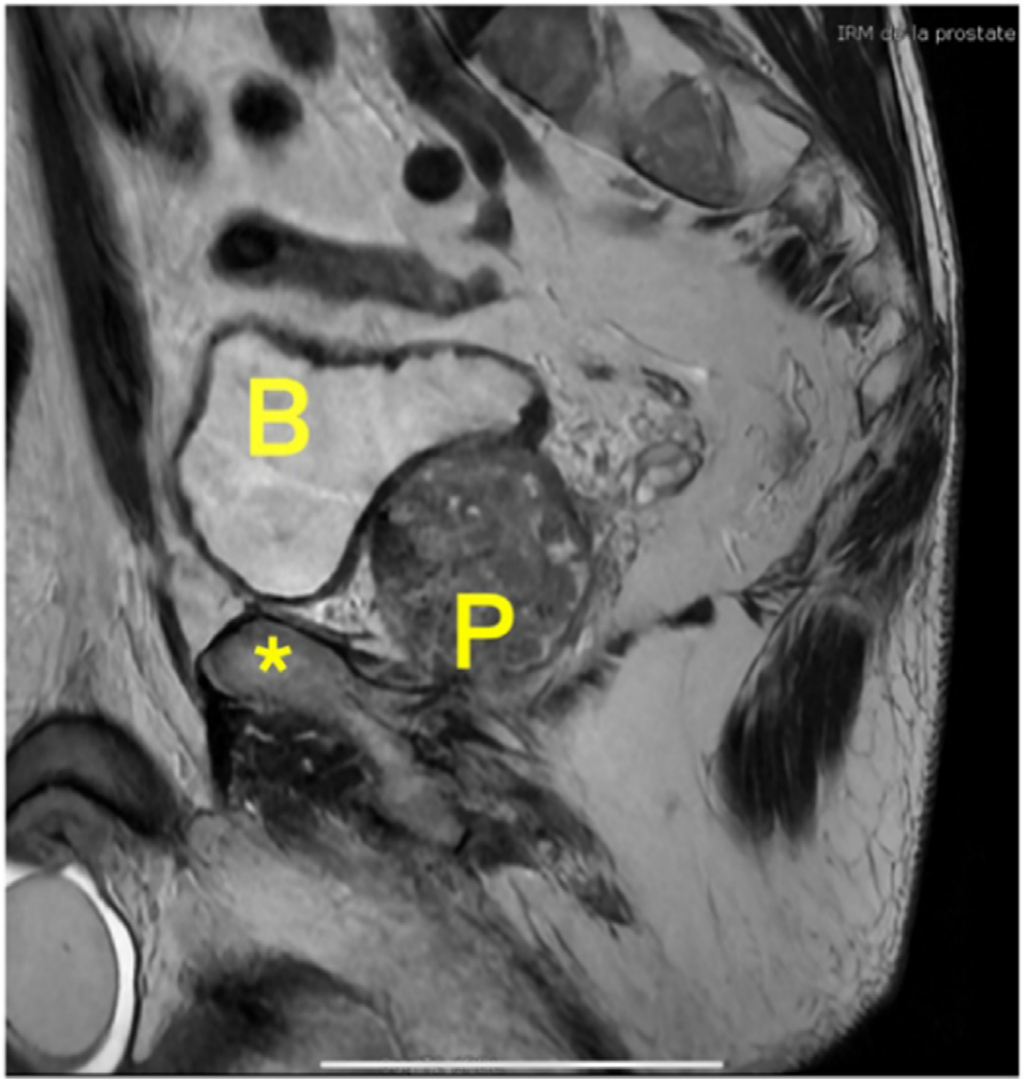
Sagittal T1 weighted MRI of the prostate showing 2 nodules with suspected capsular invasion in a potentially malignant lesion (∗ = Pubis). *B*, bladder; *P*, prostatis; *MRI*, magnetic resonance imaging.
